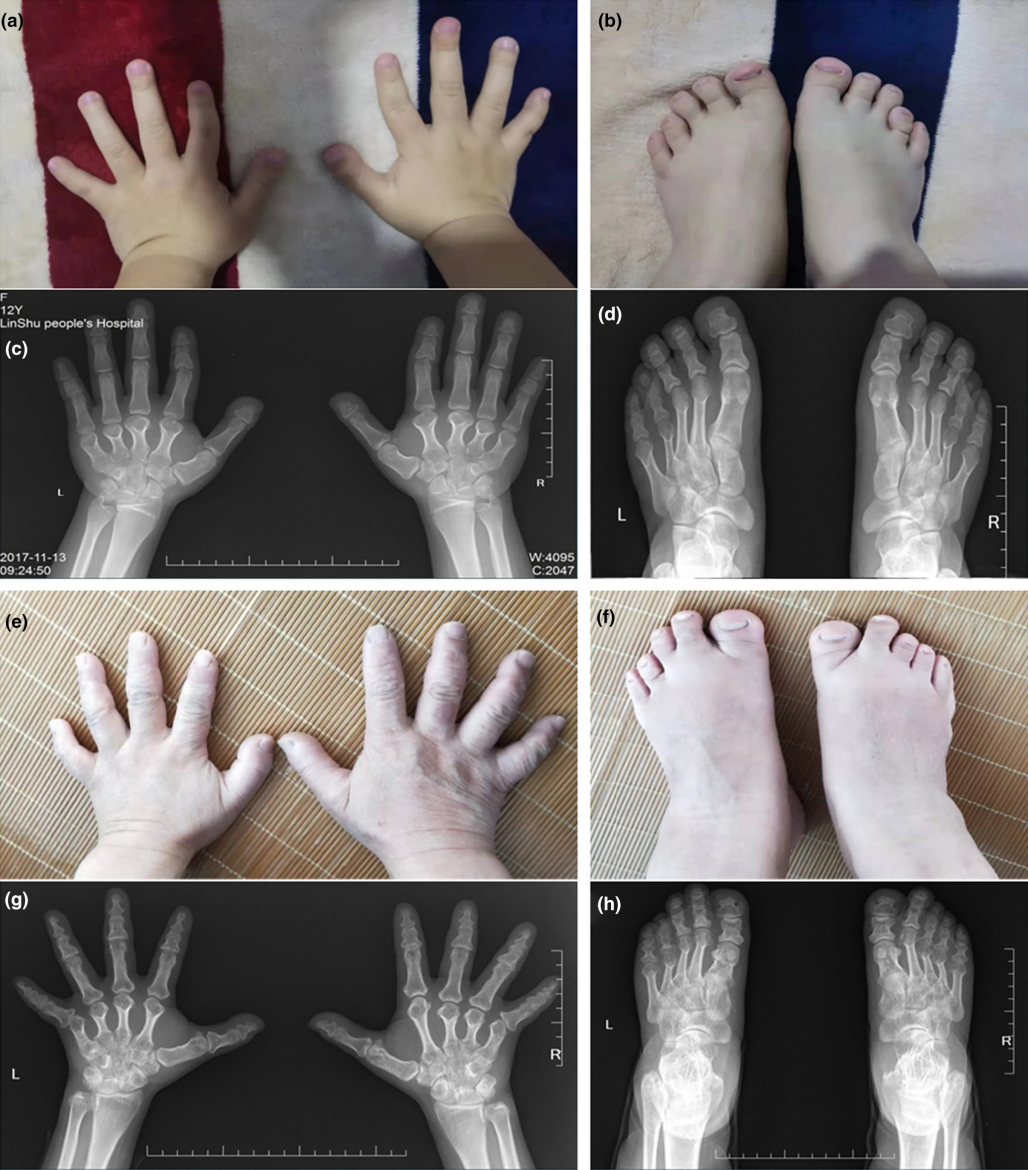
Clinical and radiological features observed in the proband (IV11) in the age of 17 (a–d) as well as III6 at the age of 32 years (e–h). (a) Clinically observed bulky and stocky hands. (b) Shortening of fourth and fifth toes. (c) Hand radiograph showing shortened metacarpals and phalanges as well as abnormal cone‐shaped epiphyses of the metacarpals. (d) Shortening fourth and fifth metatarsals of both feet. (e) Clinically observed short and stubby hands. (f) Shortening of third, fourth, and fifth toes in right foot, shortening of third, fourth in left foot. (g) Radiologically observed shortened metacarpals and phalanges as well as abnormal cone‐shaped epiphyses of the metacarpals. (h) Foot radiograph showing shortened third, fourth, and fifth metatarsals in right foot and shortened third, fourth metatarsals in left foot.
